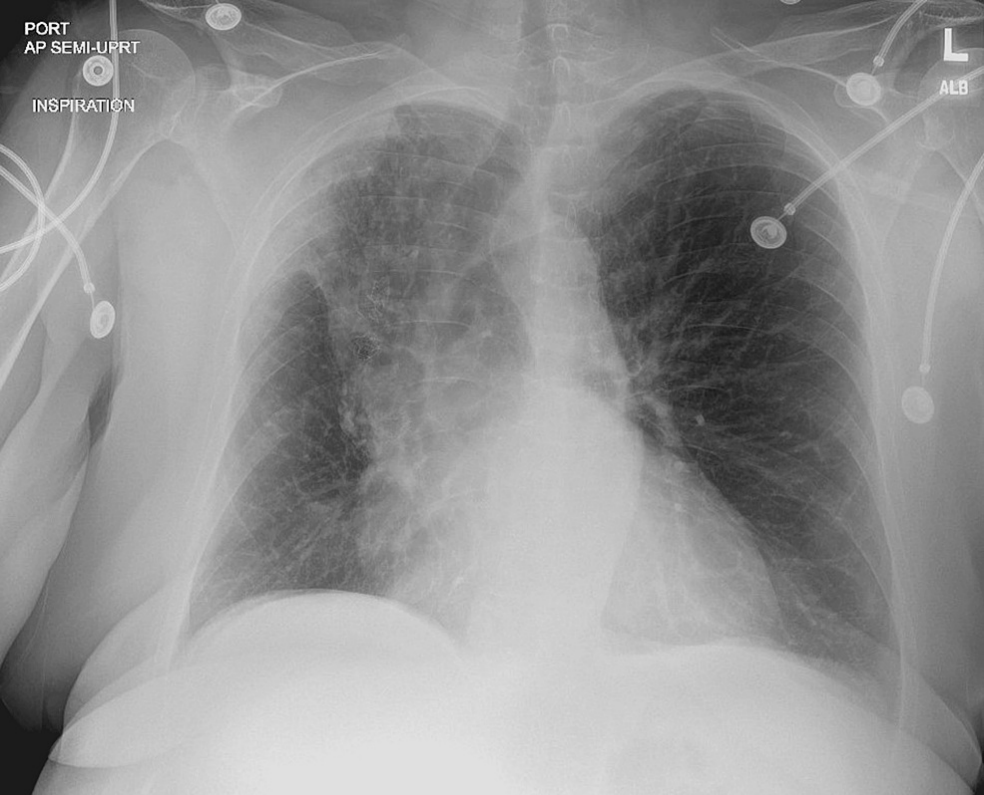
Post-procedure chest radiograph showing rapid atelectasis of the RUL and tracheal deviation to the right RUL: right-upper lobe
